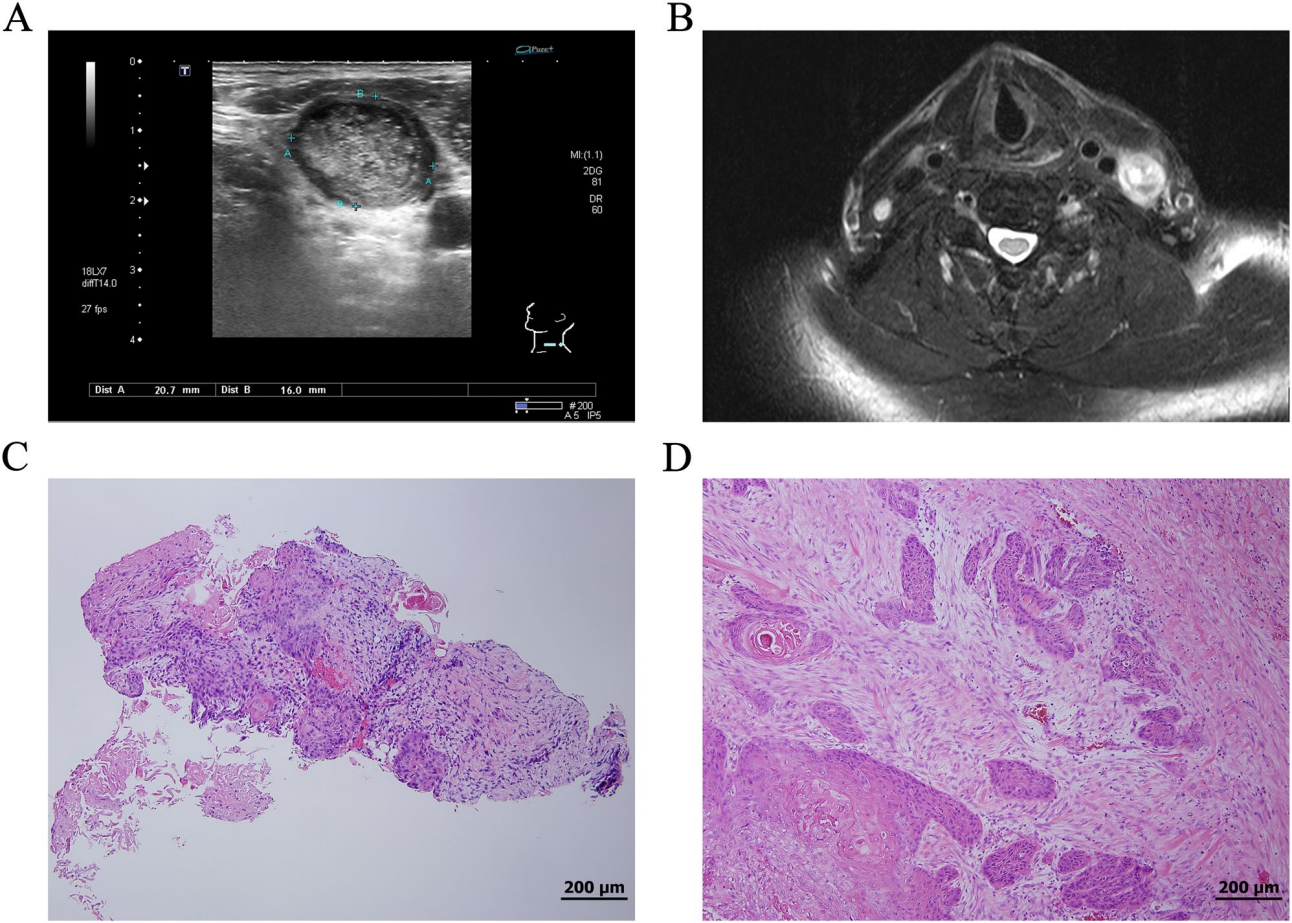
(A) Ultrasound image of the treated left tongue cancer with an ipsilateral level IV nodal mass; (B) corresponding magnetic resonance imaging showing necrotic changes with a T2 axial view; (C) core-needle biopsy (CNB) revealed necrotic tissue with metastatic squamous cell carcinoma (SCC) in nests infiltrated in the fibrotic stroma; and (D) tissue from salvage neck dissection revealed metastatic SCC without extranodal extension.
